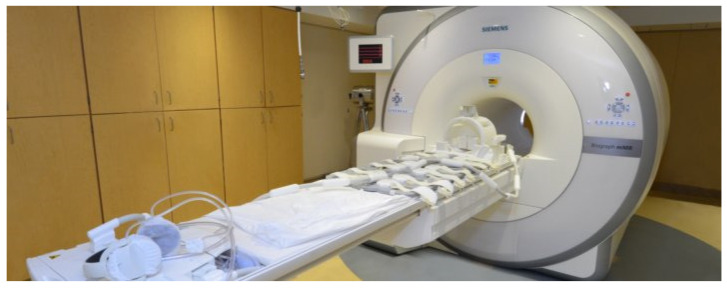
Positron Emission Tomography and Magnetic Resonance Imaging scanner; photo courtesy of the Center for Clinical Imaging Research in the Mallinckrodt Institute of Radiology at Washington University School of Medicine in St. Louis.
